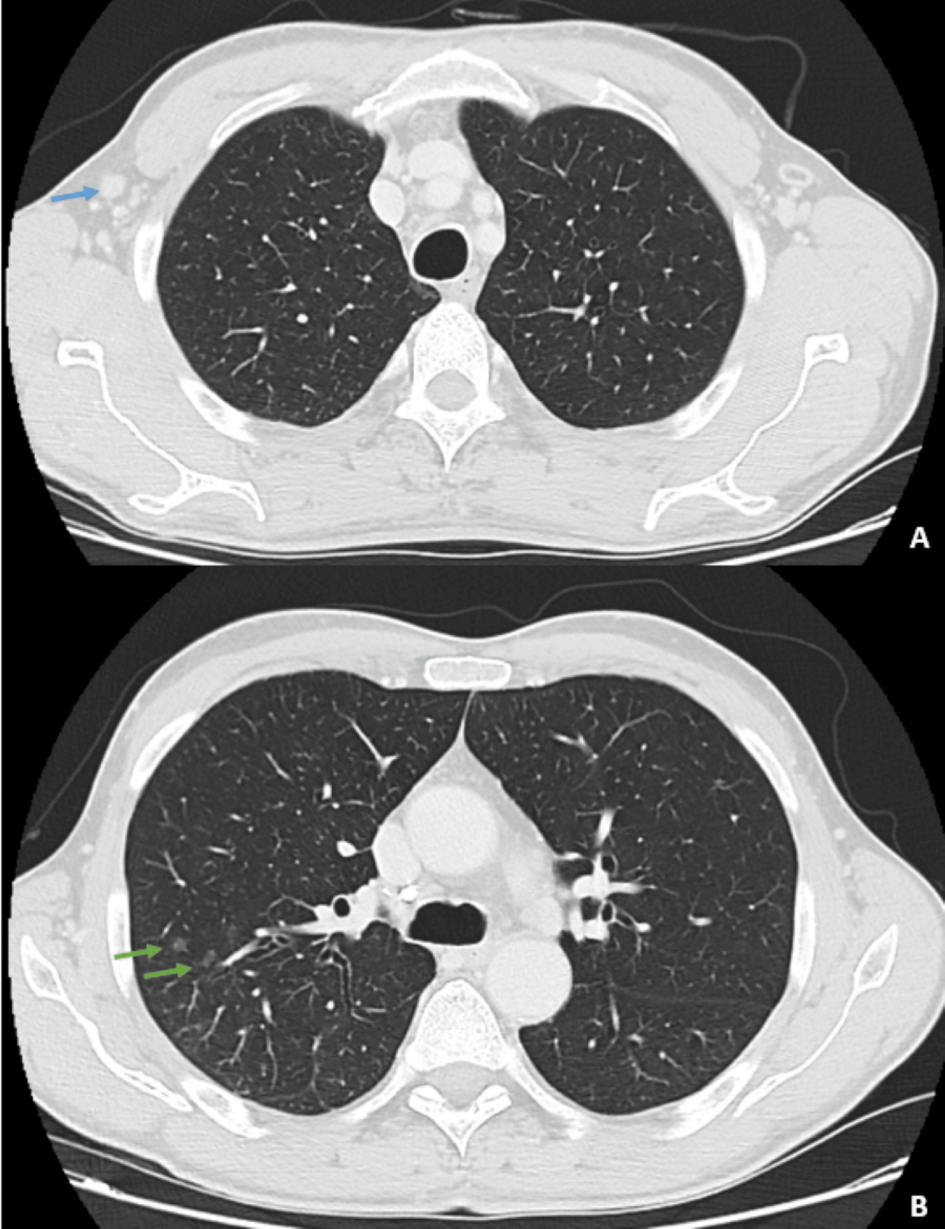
A CT scan of the chest and abdomen showed bilateral axillary lymphadenopathy (indicated by the blue arrows in A) and centrilobular micronodules in the upper lobe of the right lung (indicated by the green arrows in B).
